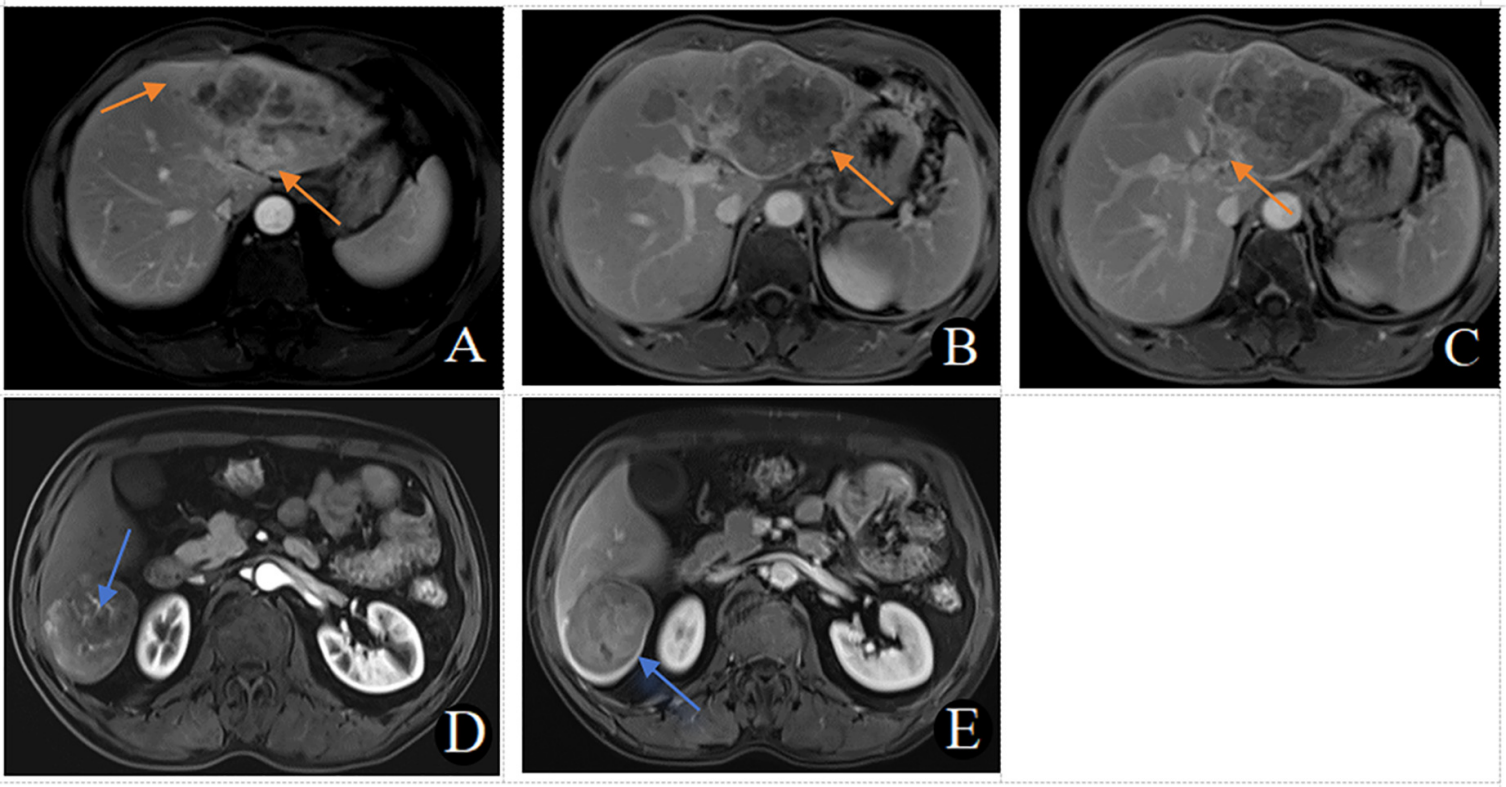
(A–C) MRI in a 56-year-old male patient with early recurrence (within 2 years) after liver cancer resection. (A) Arterial phase late peritumoral enhancement is seen (orange arrow). (B) Two intrahepatic lesions are present, with the larger lesion showing irregular tumor margins in the portal venous phase (orange arrow). (C) A filling defect in the left branch of the portal vein and the formation of a cancerous thrombus is observed in the portal venous phase (orange arrow). This patient scored 9 points. (D, E) MRI in a 54-year-old male patient without early recurrence (within 2 years) after liver cancer resection. D. No peritumoral enhancement is observed in the arterial phase, with visible intratumoral arteries. (E) The tumor margins are smooth, with uniform enhancement in the portal venous phase. This patient scored 0 points.
